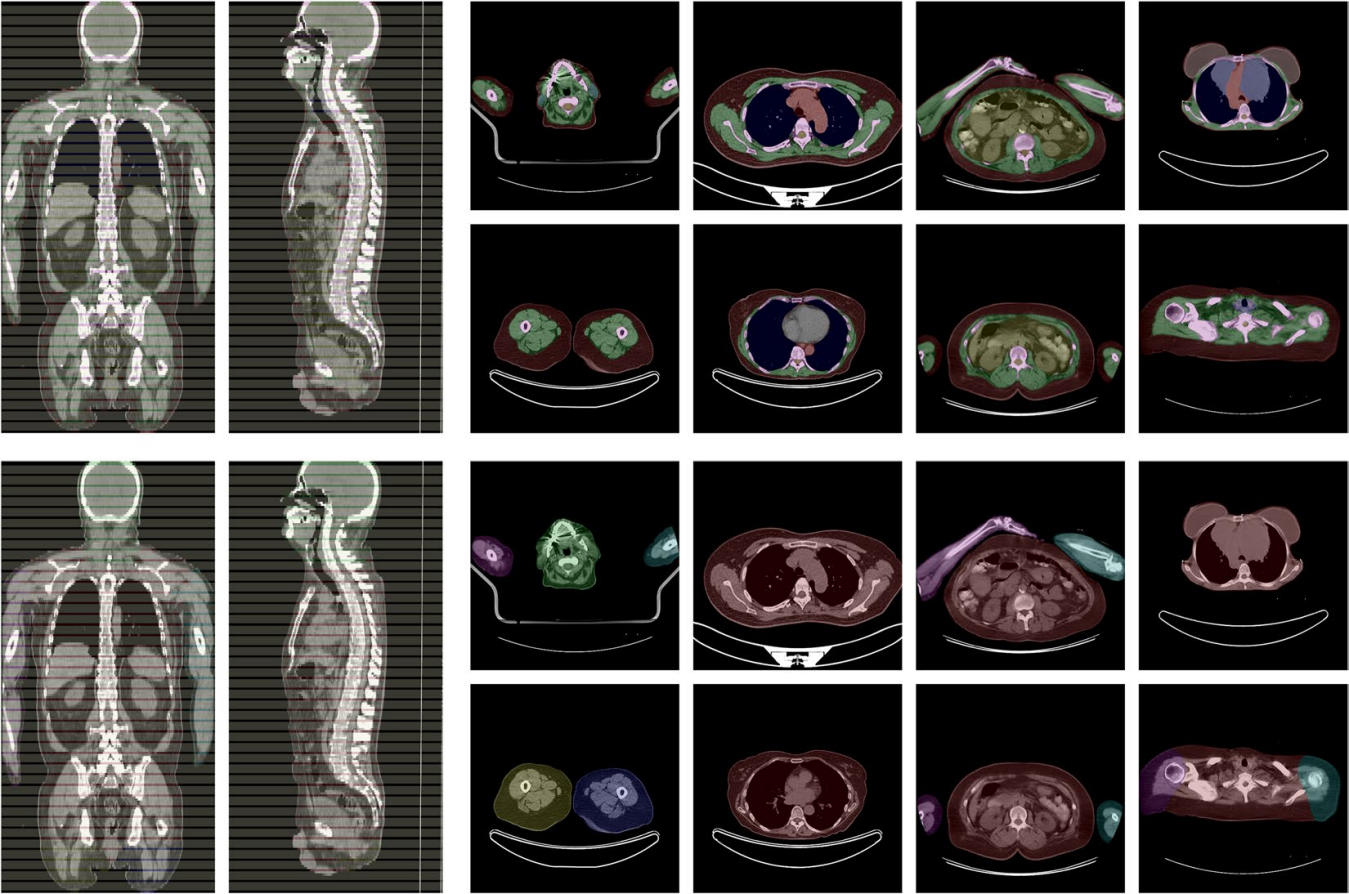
Exemplary slices of the annotated CT cases. On the left side, the sparse annotation scheme is visible in the coronal and sagittal slices, since only every fifth axial slice was annotated. On the top, the body regions label set is shown (e.g. red = subcutaneous tissue; green = muscles; blue = thoracic cavity; yellow = abdominal cavity), on the bottom, the corresponding body parts label set is shown (red = torso; yellow = right leg; blue = left leg; green = head; pink = right arm; cyan = left arm).
